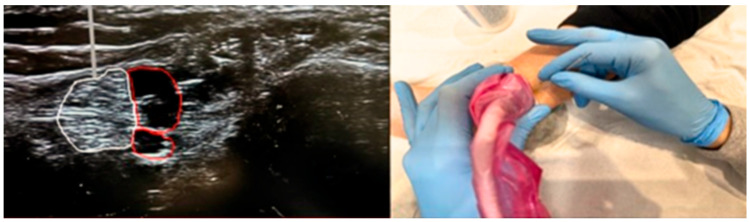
Ultrasound image demonstrating the anatomical localization of the posterior tibial nerve at the level of the medial ankle. The figure includes the appropriate placement and orientation of the ultrasound probe, as well as the in-plane approach typically used for optimal visualization of the nerve (white) and surrounding structures. Additionally, the hypoechoic network observed in the image corresponds to the vascular bundle (red), which accompanies the nerve and includes the posterior tibial artery and vein.
